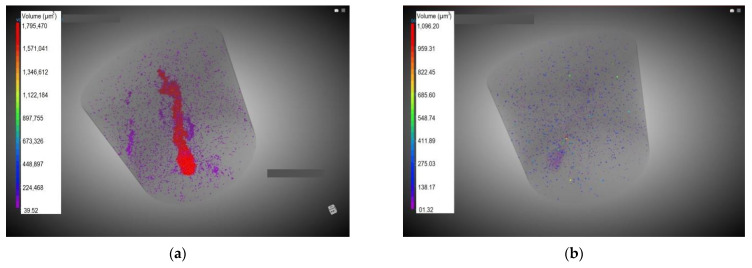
Images obtained from sample 1 CT: (a) cracks and pores (colour-coded bar ranges from 39.5 to 1.79 × 10^6^ µm^3^); (b) white particles (colour-coded bar ranges from 1.3 to 1096.2 µm^3^).
